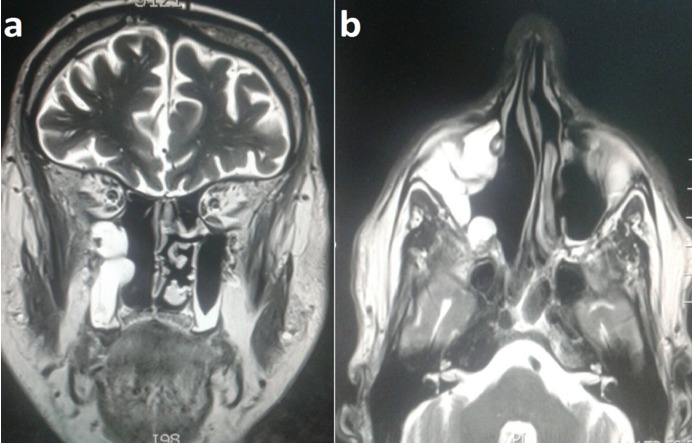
The post-treatment magnetic resonance imaging showed retention in the right maxillary sinus without suspicious mass detected
